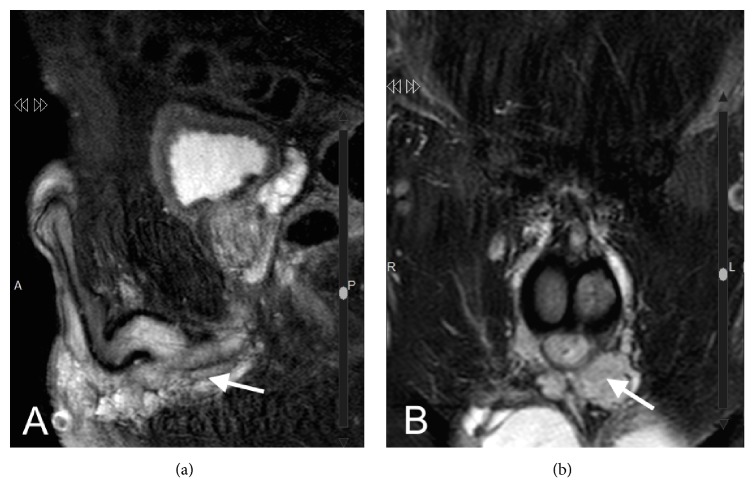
MRI images in T2 sequence, showing periurethral hemangioma. (a) Sagittal section. (b) Coronal section.
